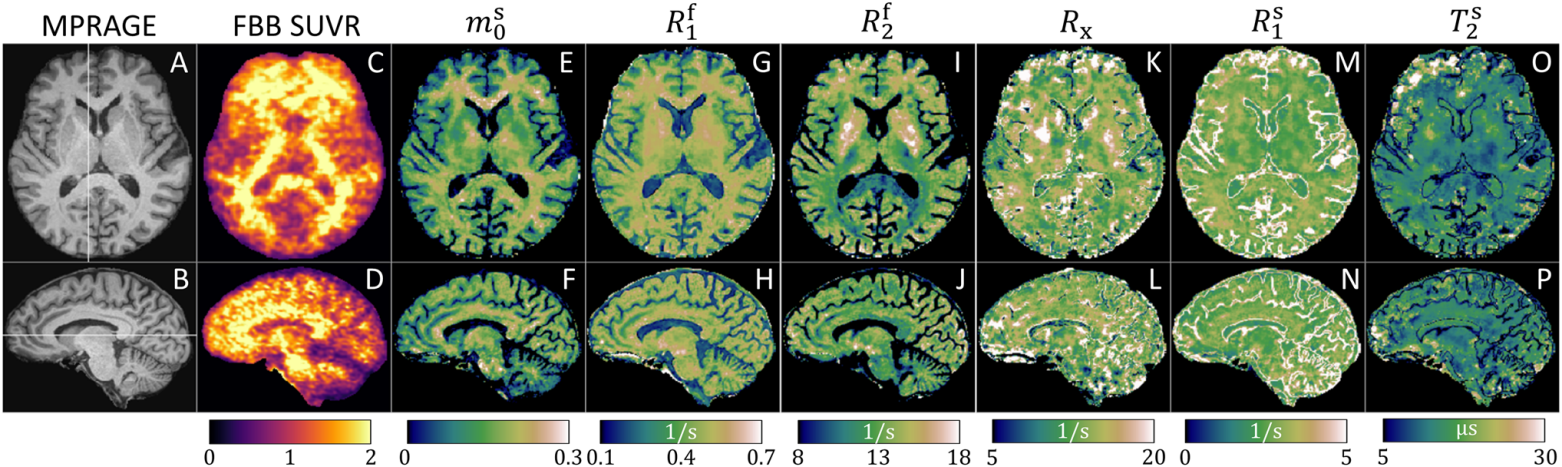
Exemplary MPRAGE (A–B), [^18^F]florbetaben (FBB) PET SUVR(C–D), and quantitative magnetization transfer maps (E–P)in a cognitively normalAβ+participant. The six qMT parameters are the semisolid pool size$m_{0}^{s}$,the relaxation rates$R_{1,2}^{\text{f},\text{s}}=1\text{​}/T_{1,2}^{\text{f},\text{s}}$(where the superscripts^f^and^s^denote the free and semisolid spin pools, respectively), and theexchange rate$R_{\text{x}}$. The MPRAGE is used for FreeSurfer-based corticalparcellation and calculation of cortical thicknesses. The FBB SUVRimages are used to compare the qMT parameters with a relativeconcentration of Aβ.
